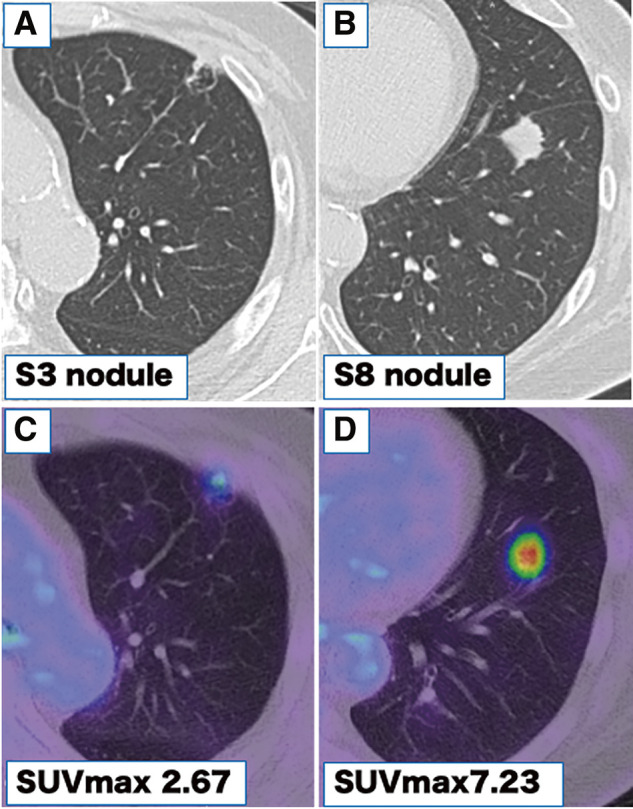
Chest computed tomography of the nodules. (A, B) Nodules measured 1.1 × 0.8 cm and 1.8 × 1.7 cm in the left S3 (A) and S8 segments (B), respectively. (C, D) 18F-fluorodeoxyglucose positron emission tomography demonstrated abnormal uptake in the tumors, with maximum standardized uptake values of 2.67 (C) and 7.23 (D), respectively.
